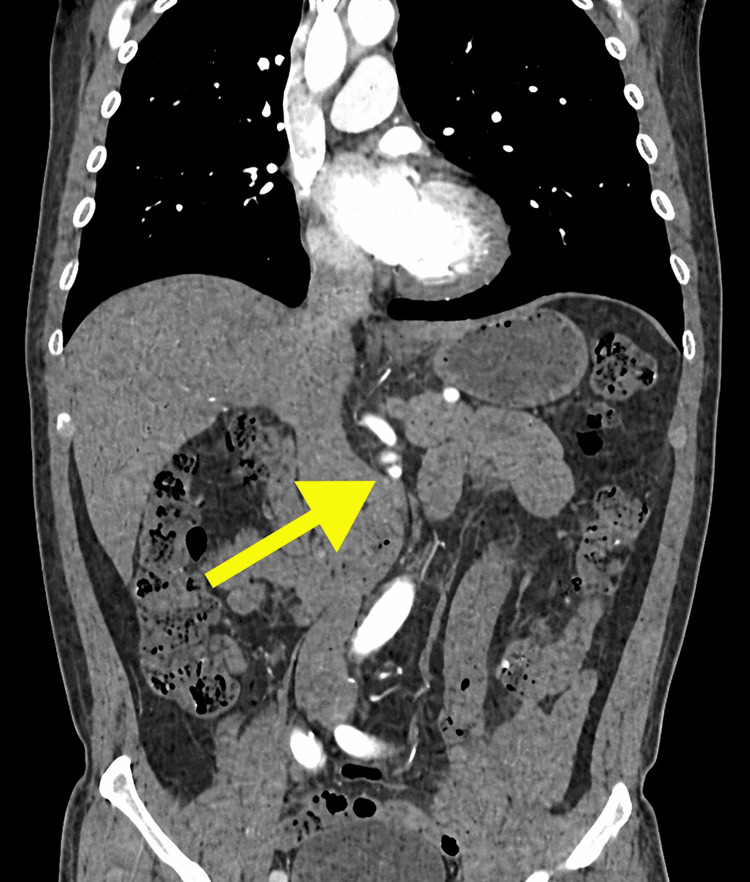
Coronal reformatted CT image of the SMA. A linear hypodense intimal flap (arrow) is visualized, clearly separating the true and false lumens. CT, computed tomography; SMA, superior mesenteric artery
